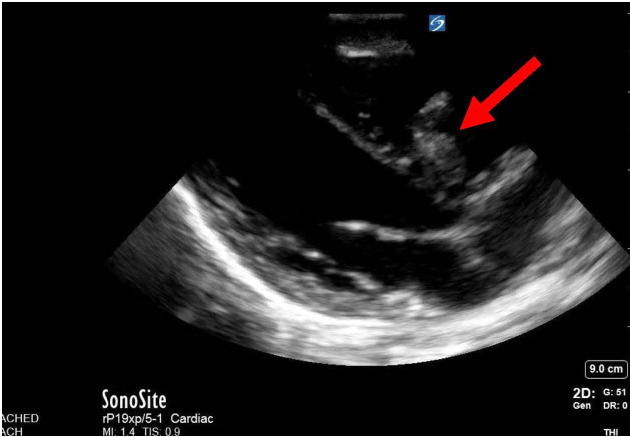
This is a still image of a parasternal long view of the heart. Overall, there are some irregularities in the basal aspect of the inter-ventricular septum and thickness to one of the leaflets of the tricuspid valve.
